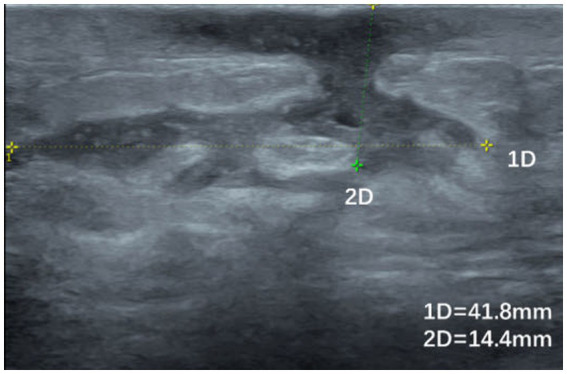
Ultrasound image of a patient with GLM with hypoechoic areas leading to the skin and sinus tract formation.
